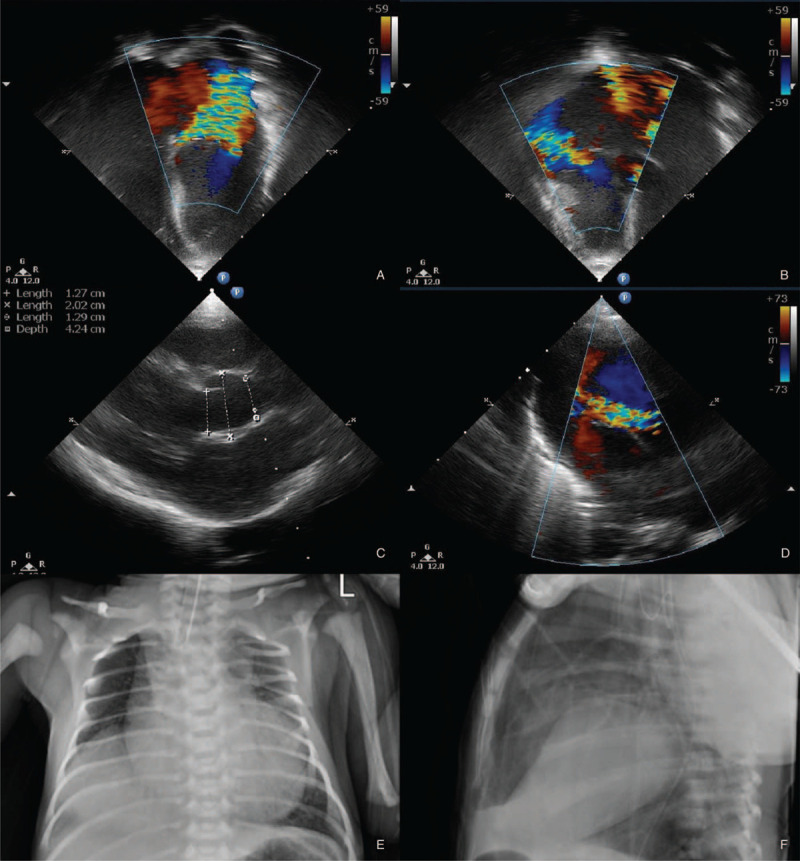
Echocardiography results showing severe mitral (A) and moderate tricuspid regurgitation (B) on 4-chamber views. Aortic root dilatation (C) on parasternal long axis view and moderate aortic regurgitation (D) on five-chamber view. X-ray suggesting liver herniation (C, D).
